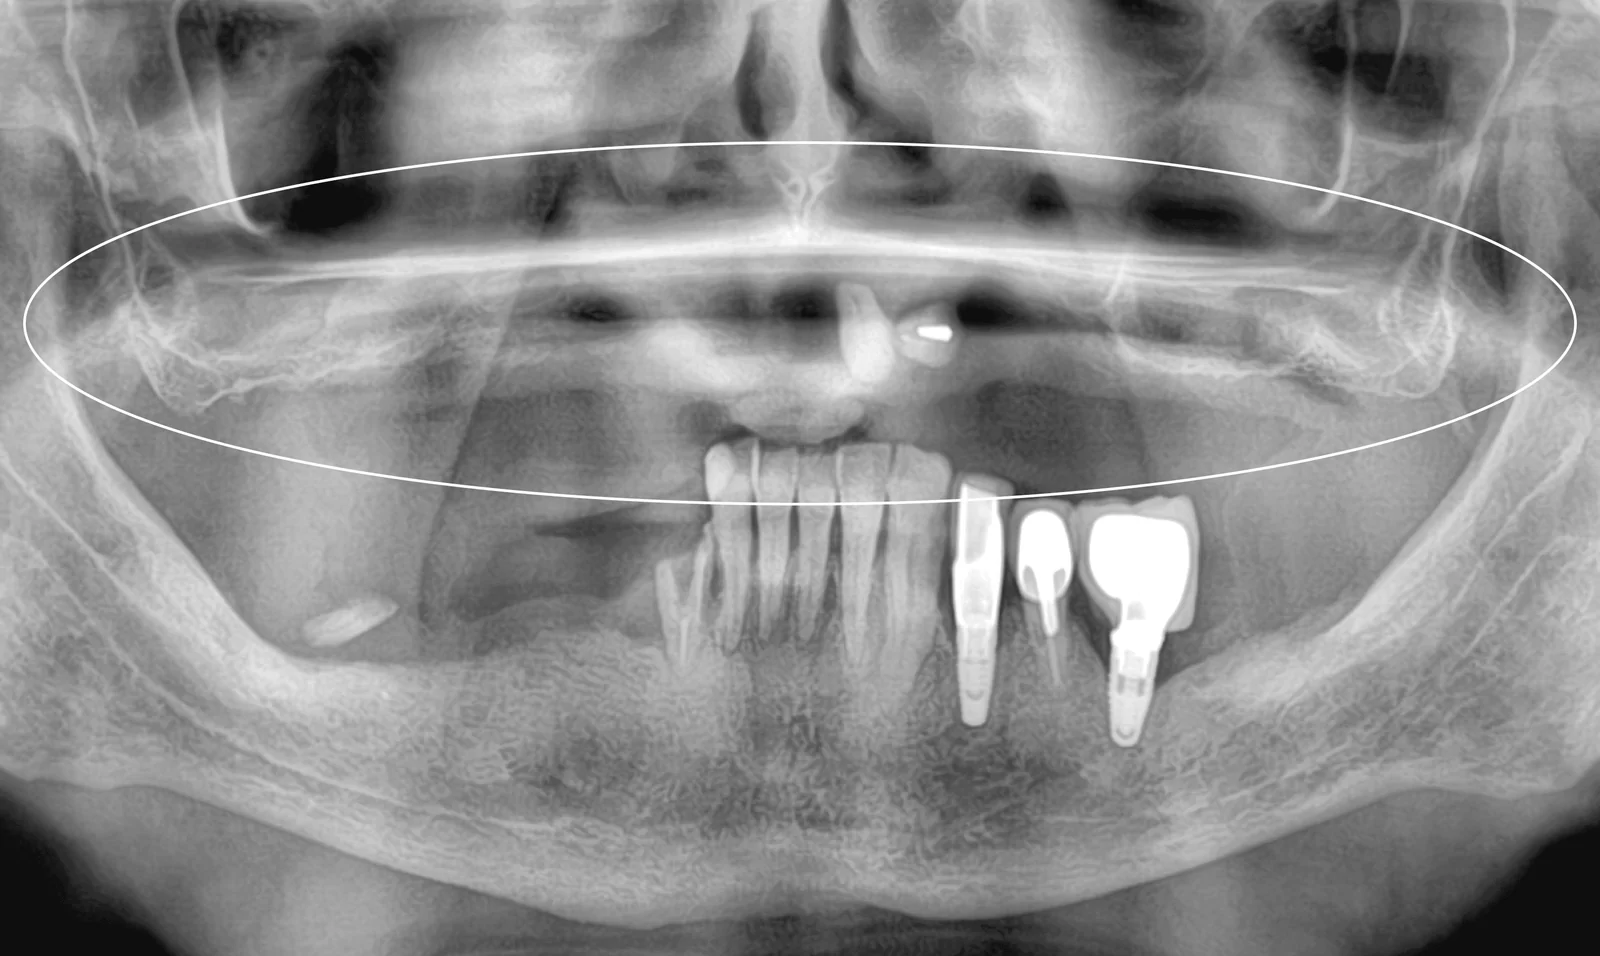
Preoperative panoramic X-ray. The white oval delineates the extent of the residual maxillary bone loss and the area of extreme maxillary atrophy (Cawood–Howell Class VI).
